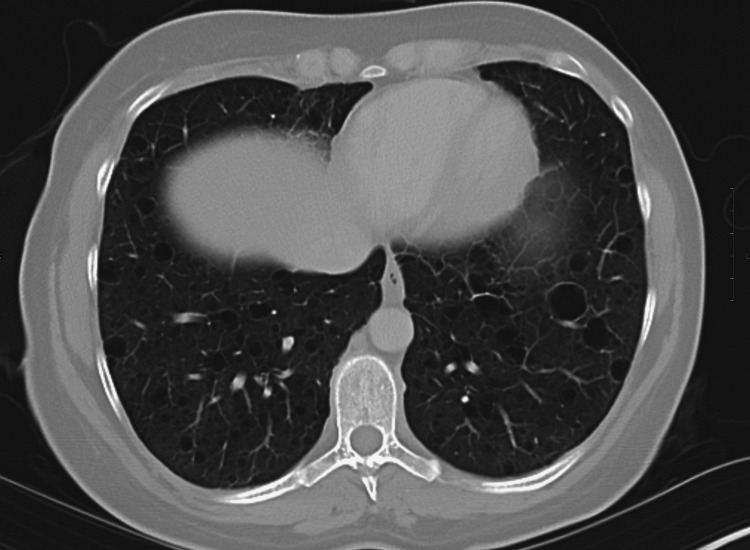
Contrast-enhanced CT of the lower lung zones showing numerous bilateral discrete cystic spaces with very thin or imperceptible walls Lung volumes are normal with no evidence of emphysema. CT: computed tomography
